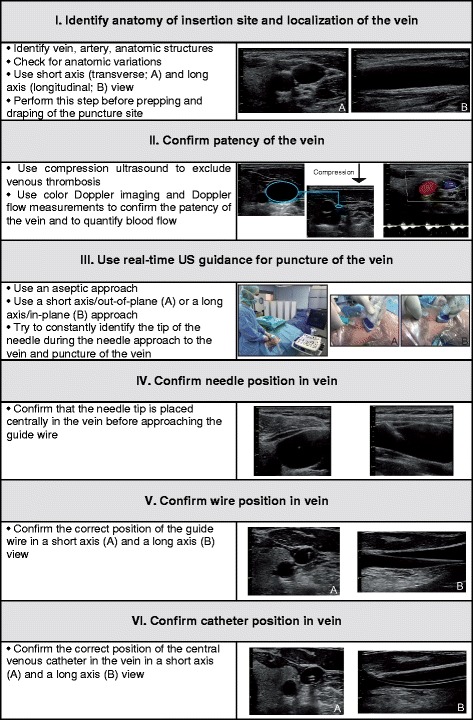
Six-step approach to ultrasound-guided central venous catheter placement
